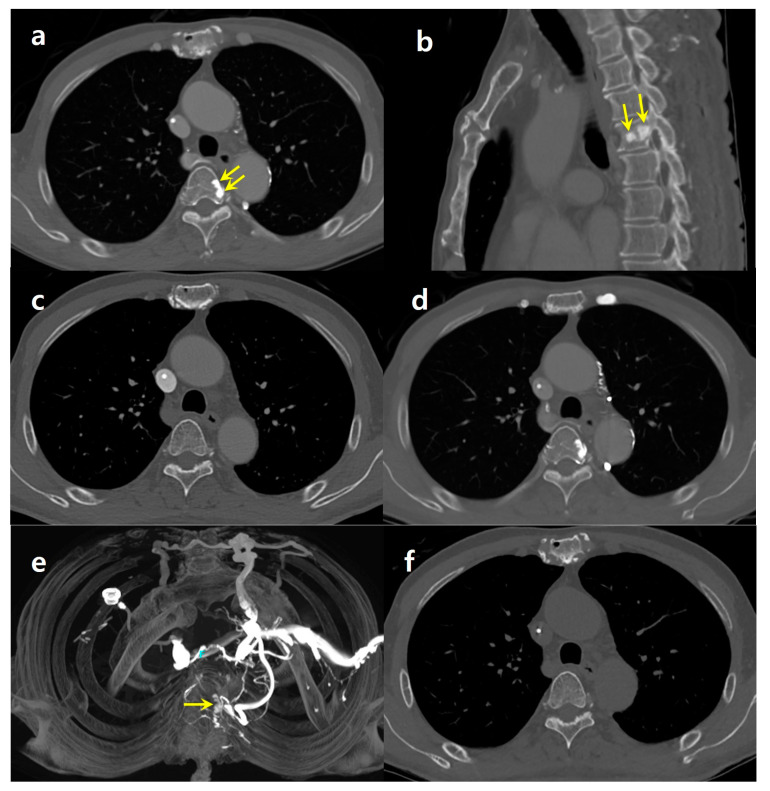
A 69-year-old male with gall bladder cancer. (a) An axial contrast-enhanced chest CT image with left-side contrast injection reveals a focal sclerotic area on the left side of the T4 vertebral body (arrows). A chemoport catheter is inserted in superior vena cava. (b) Sagittal reformatted image shows nodular sclerotic foci at T4 (arrows). (c) Chest CT image obtained three months ago with right-side injection does not show the sclerotic lesion. (d) Chest CT image obtained six months ago with left-side contrast injection shows a focal sclerotic area in the same location. (e) Maximum-intensity projection (MIP, d) image from enhanced CT with left-side injection demonstrates a focal enhancing area at T4 (arrow), with the surrounding vertebral venous plexus opacified through reflux from the left brachiocephalic vein. Collateral flows are also noted in both internal mammary and left superior intercostal veins. (f) A follow-up chest CT with contrast injection through his right arm showing no evidence of a sclerotic lesion in T4 or paravertebral venous collaterals.
